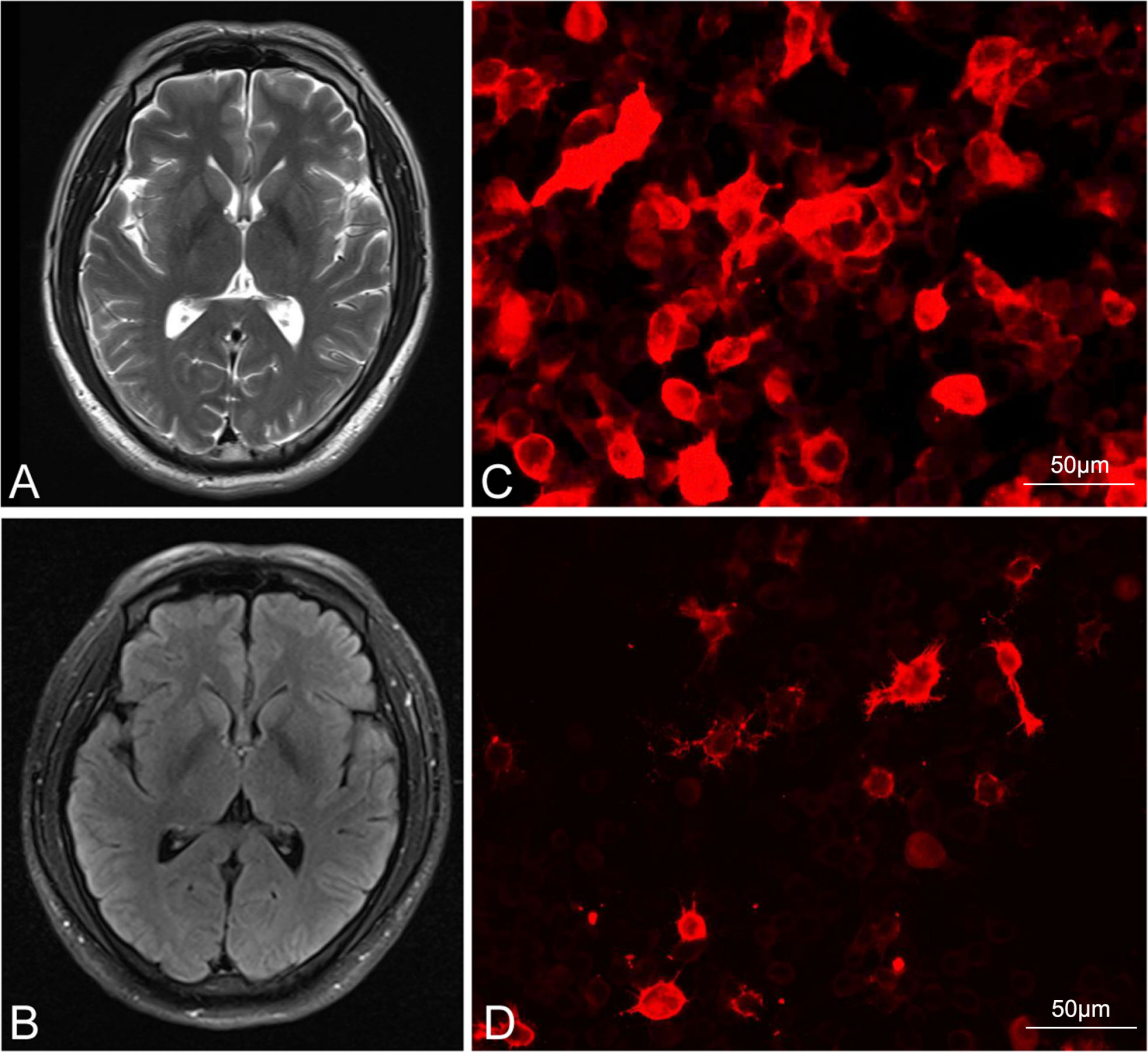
Brain imaging and serum anti-LGI1 results for patient 2, and serum anti-NMDAR results for patient 3. (A, B) show the lack of abnormal signaling on MRI for patient 2. (C, D) show serum positivity for the LGI1 antibody and anti-NMDAR in patients 2 and 3, respectively (Bar: 50 μm). LGI1, leucine-rich glioma-inactivated 1; NMDAR, N-methyl-D-aspartate receptor; MRI, magnetic resonance imaging.
